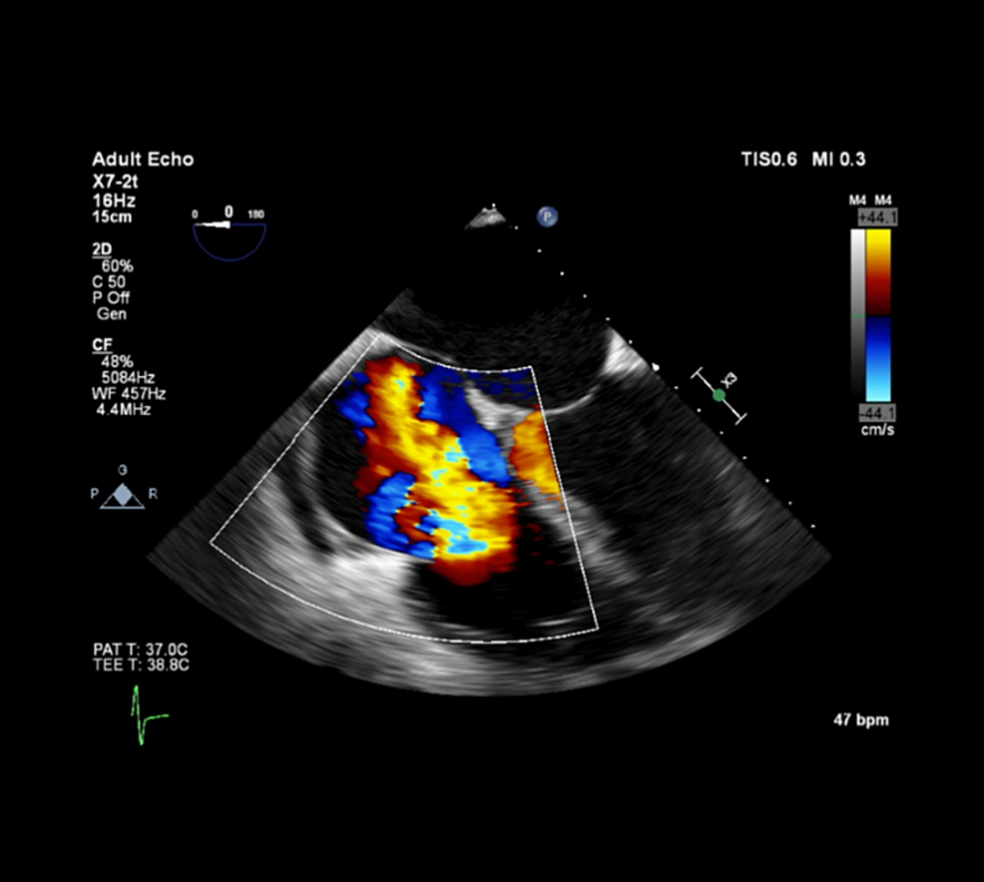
Transesophageal echocardiography (TEE) image demonstrating severe tricuspid regurgitation (TR) on initial presentation for liver transplantation (mid-esophageal 4-chamber view).
